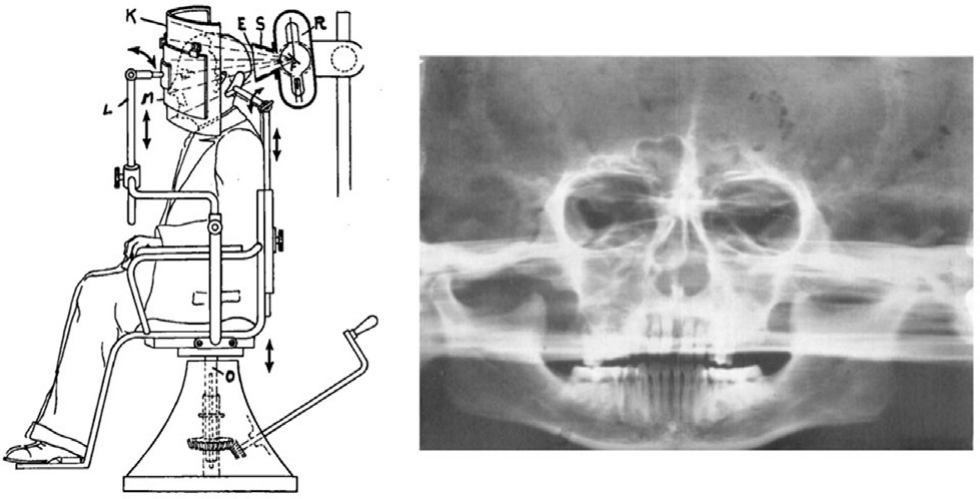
First image of rotational panoramic tomography Note. Reprinted from “A new tomographical method for radiographing curved surfaces”, by Paatero YV.,1949, Acta Radiol 1949; 32:180, Figure 2.
